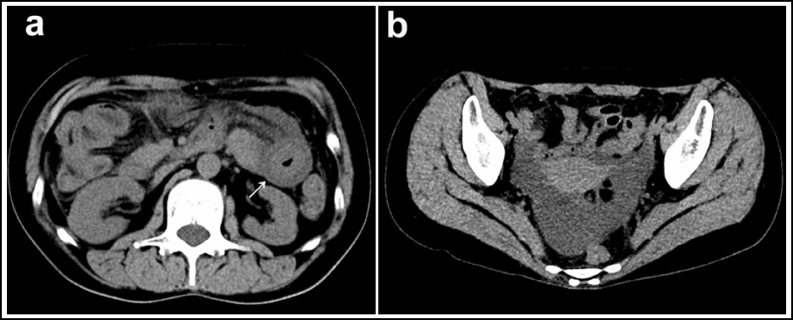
CT images of the patient. Computed tomography scan of the abdomen showing circumferential edema of the proximal jejunal bowel (arrow-head) and significant thicken of the mesenterium (a), together with a moderate amount of ascites (b).
